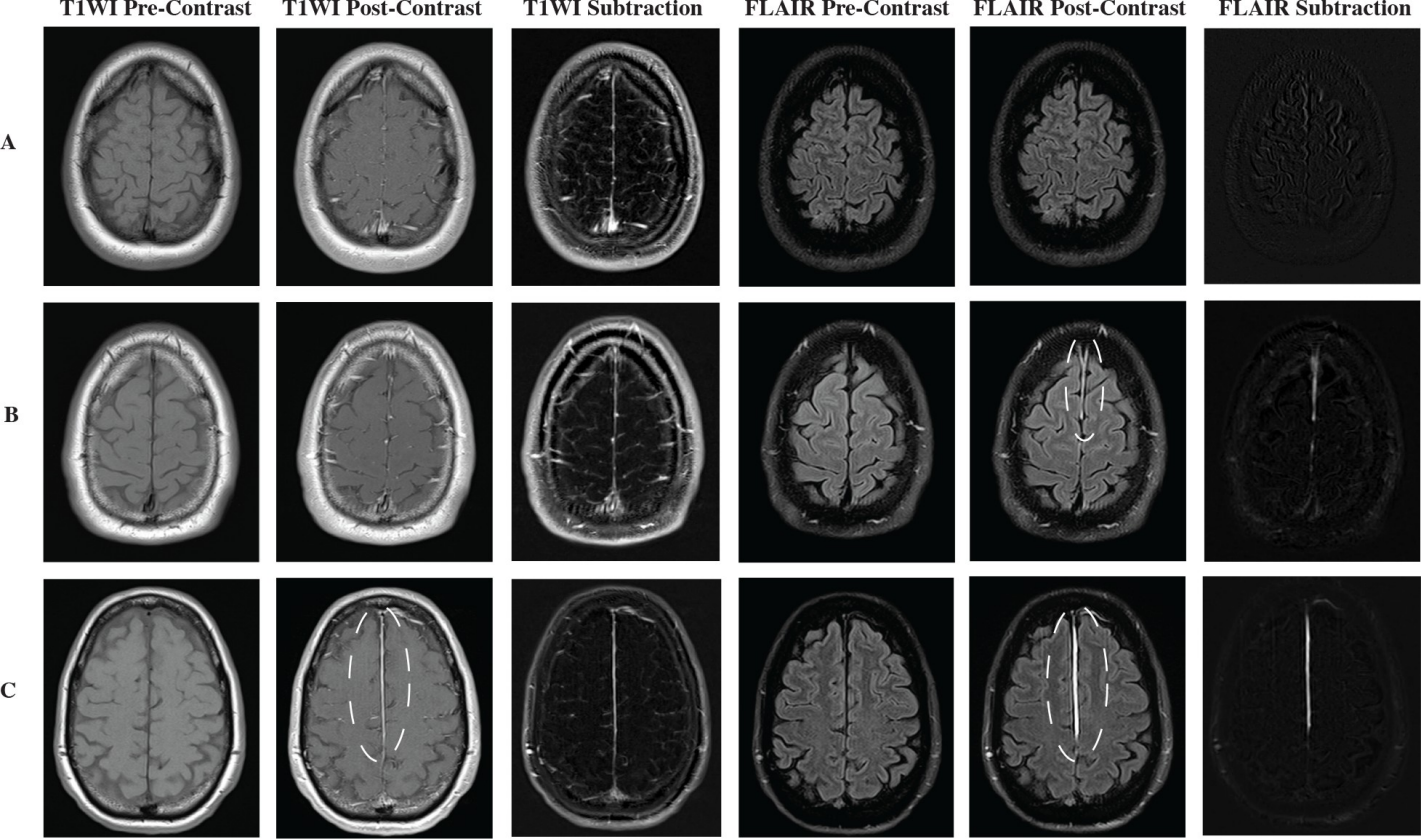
Examples of subjects studied in this cohort with possible scoring combinations by blinded readers. (A) Sets of T1WI and FLAIR pre and post-contrast of a subject scored as negative all around by all readers on both sets of sequences. (B) Sets of T1WI and FLAIR pre and post-contrast for a subject scored as positive for traumatic meningeal enhancement only on FLAIR sequence, as denoted by the dotted line. Traumatic meningeal enhancement is most conspicuous in the FLAIR post-contrast scan in both the falx cerebri and right convexity. (C) Pairs of T1WI and FLAIR pre and post-contrast scored as positive for traumatic meningeal enhancement on both sequences. Traumatic meningeal enhancement is denoted by the dotted line and is visualized in the falx cerebri alone on both post-contrast scans. The subtraction images visually represent the calculated differences between the pre-contrast and post-contrast T1 or FLAIR images, respectively. The T1 subtraction displays a vascular pattern not specific to meningeal enhancement, while the FLAIR subtraction primarily shows TME when it is present.
